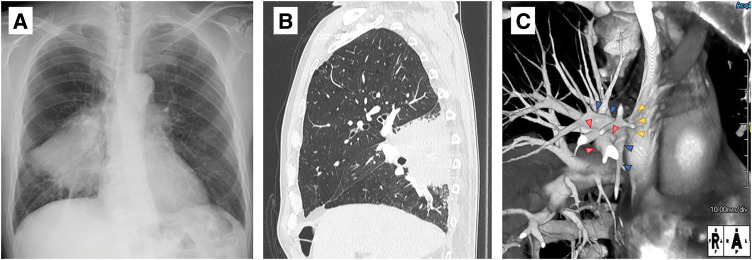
(A) Chest radiograph demonstrated a mass in the right hilar region. (B) Chest CT revealed an 8-cm mass in the right lower lobe. (C) Chest contrast CT revealed PAPVC, with the right upper lobe vein (blue arrow heads) draining into the SVC. The pulmonary artery and the point where the pulmonary vein flows into the SVC are visible (red and yellow arrow heads, respectively). PAPVC, partial anomalous pulmonary venous connection; SVC, superior vena cava
